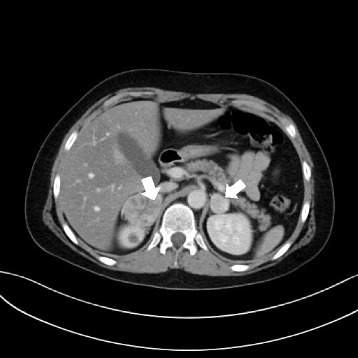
Bilateral nodular lesions in the right and left adrenal glands (phaeochromocytoma) in abdominal computed tomography
